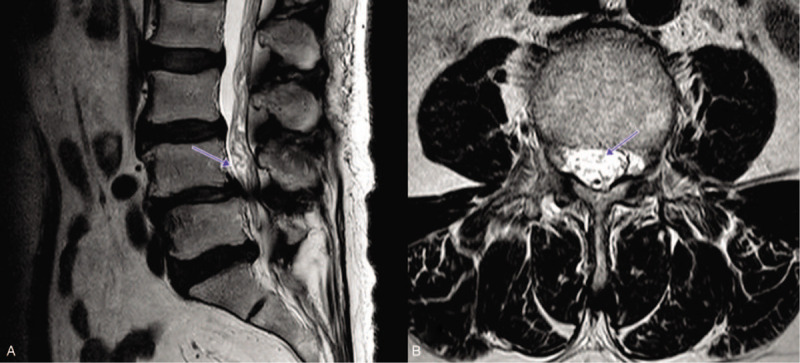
Elongated and tortuous nerve roots can be identified on sagittal (A) and axial (B) T2-weighted magnetic resonance imaging.
